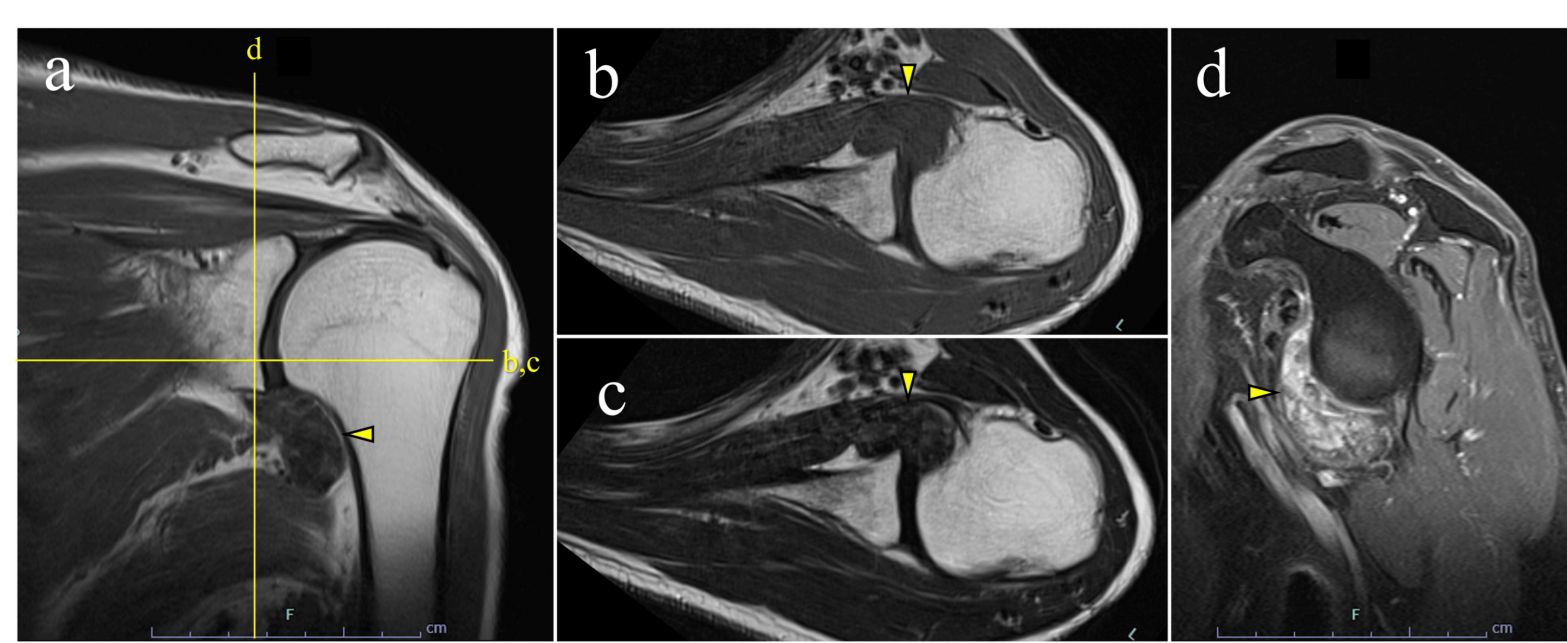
Magnetic resonance imaging findings at presentation. (a) Coronal proton density–weighted image demonstrating an intra-articular mass in the axillary pouch (arrowhead). No apparent rotator cuff tear was observed. (b) Axial T1-weighted image and (c) axial T2-weighted image showing a well-defined intra-articular mass with low signal intensity on both sequences, suggestive of a fibrous tumor (arrowheads). (d) Axial contrast-enhanced fat-suppressed T1-weighted image demonstrating heterogeneous internal enhancement of the lesion (arrowhead).
